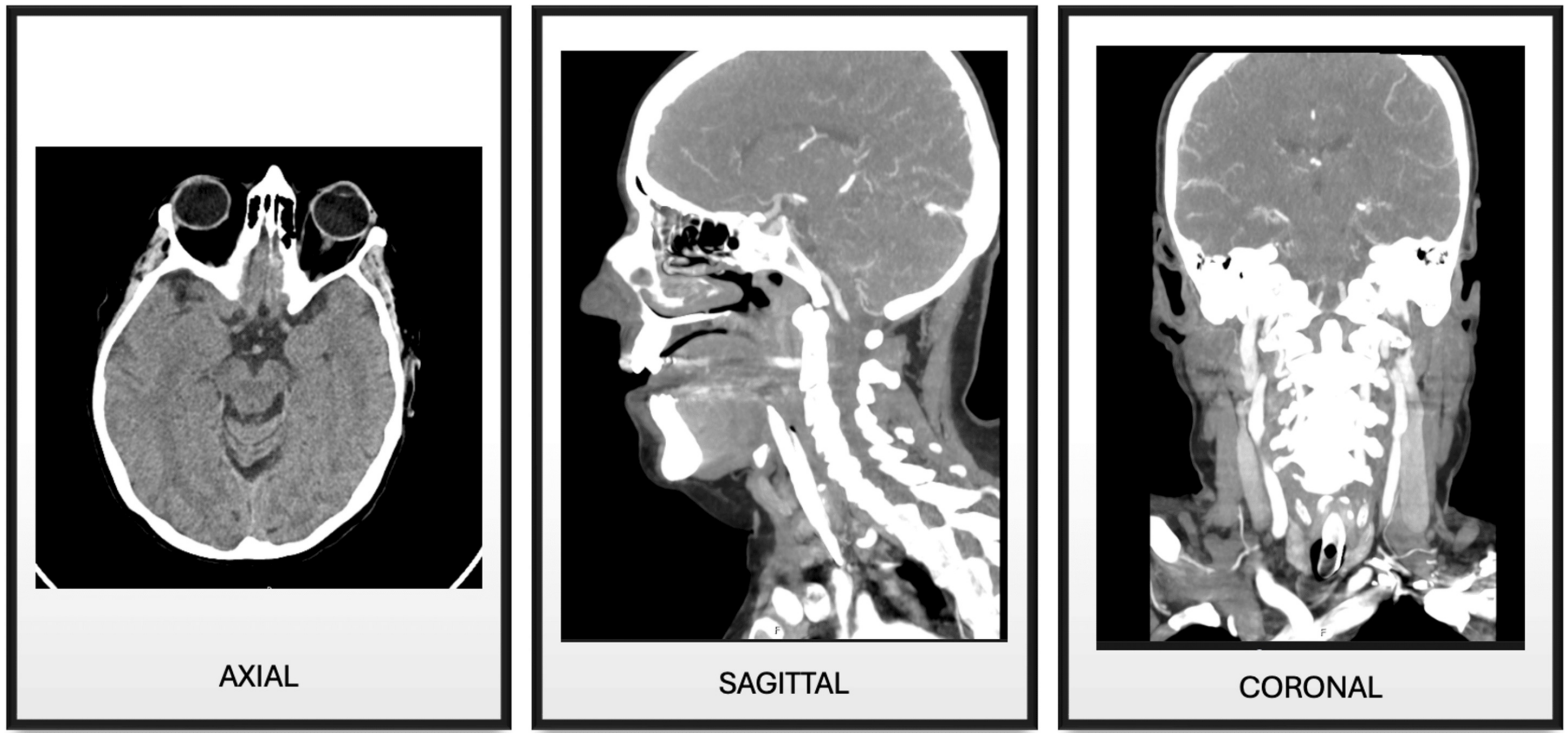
Cranial and cervical computed tomography angiography. No intracranial abnormalities were observed on density-based imaging. No evidence of foramen magnum crowding. The vascular assessment revealed normal patency of the major cervical vessels and skull base arteries.
